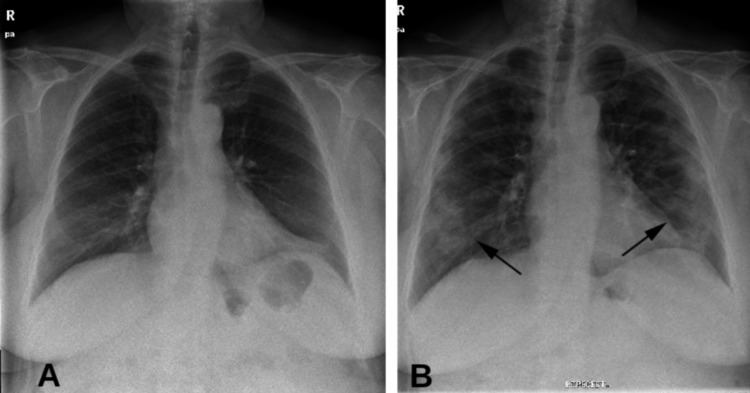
(A) A normal chest X-ray. (B) Peripheral ground-glass opacity (arrows) suggestive of SARS-CoV-2 pneumonia.
